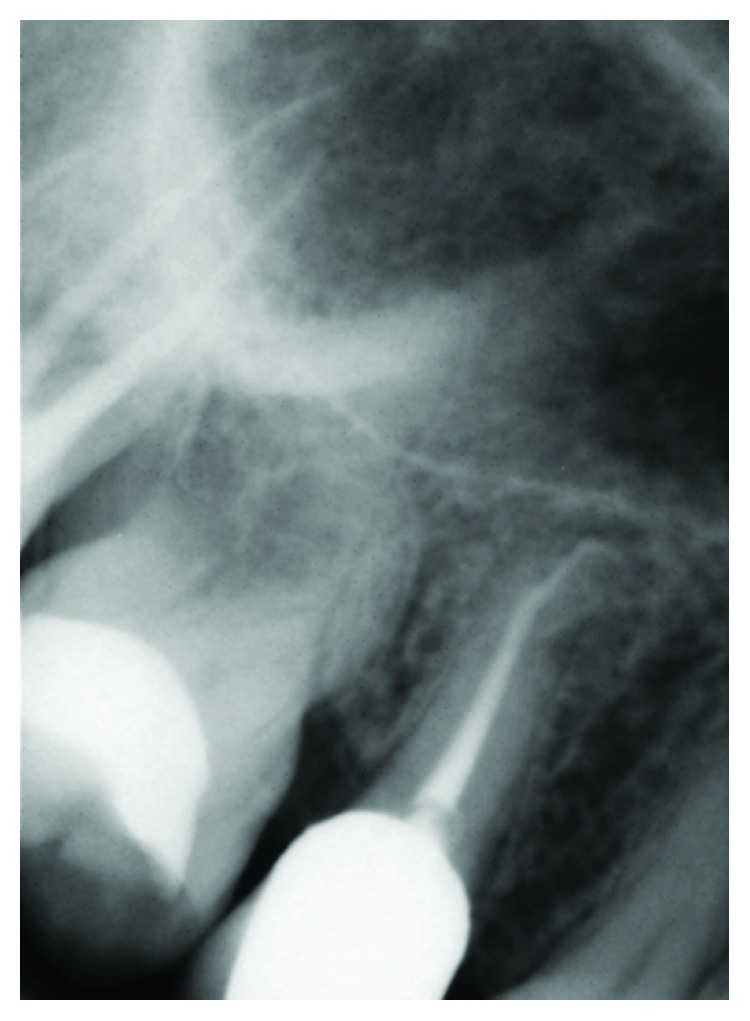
Periapical radiograph showing immediate outcome of nonsurgical endodontic retreatment performed at our dental office.
